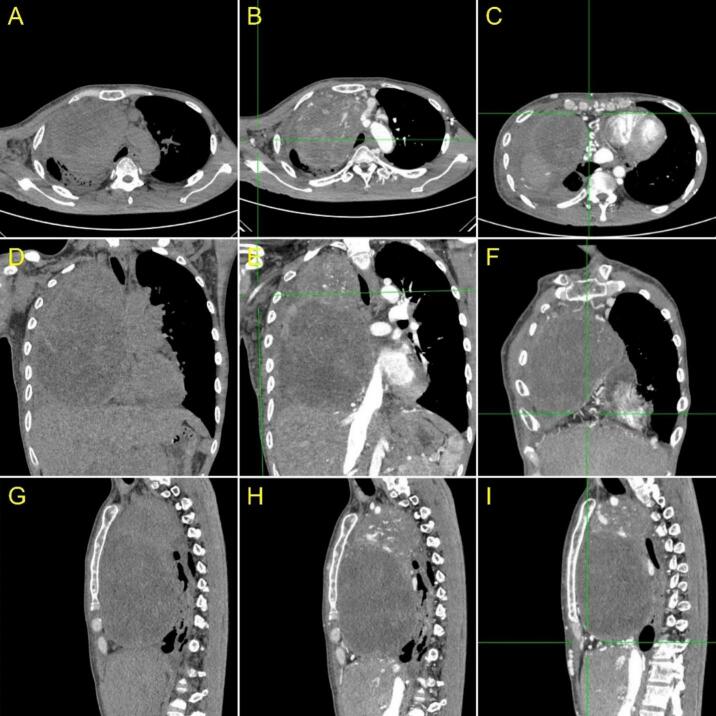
A CT-Scan of the thorax. (A, D, G) Pre-contrast axial, sagittal, coronal CT scan thorax and (B, E, H) axial, sagittal, coronal CT Scan Thorax with contrast showed a solid mass with a necrotic component in the anteromedioposterior mediastinum where contrast enhancement was visible, and (B,E) there was enlargement of right axilla (green line area). A mass appeared to encase the right pulmonary artery and vein, and the superior vena cava accompanied by (C, F, I) Collateral veins (+) (green line area).
